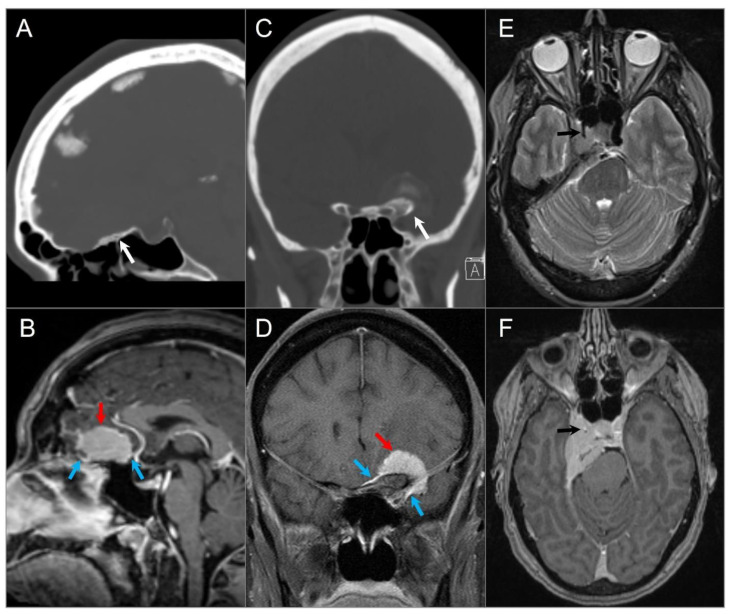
Meningiomas in three different patients. (A) Sagittal CT and (B) sagittal postcontrast T1W MR images demonstrate a homogenously enhancing extra-axial mass lesion (red arrow) at the anterior cranial fossa/planum sphenoidale with small dural tails (blue arrows) and hyperostosis (white arrow). (C) Coronal CT and (D) coronal postcontrast fat-saturated T1W images show an anterior clinoid process meningioma (red arrow) with dural tails (blue arrows), hyperostosis (white arrow), and tumoral calcification. (E) Axial T2W and (F) axial postcontrast T1W images reveal a right cavernous sinus meningioma extending through the petroclival dura, tentorium, and paraclinoid dura. The mass completely encases the right ICA with significant narrowing (black arrows).
